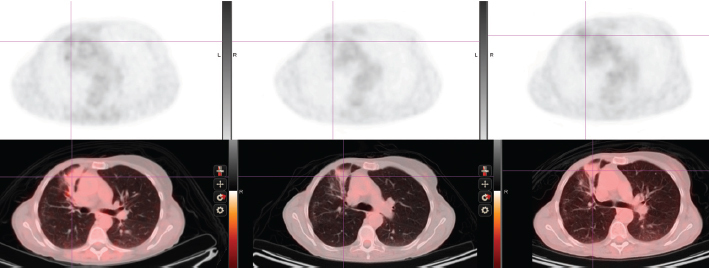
Thoracic cross-section showing right lung infiltrate and FDG uptake (upper panel). See text for time points.
